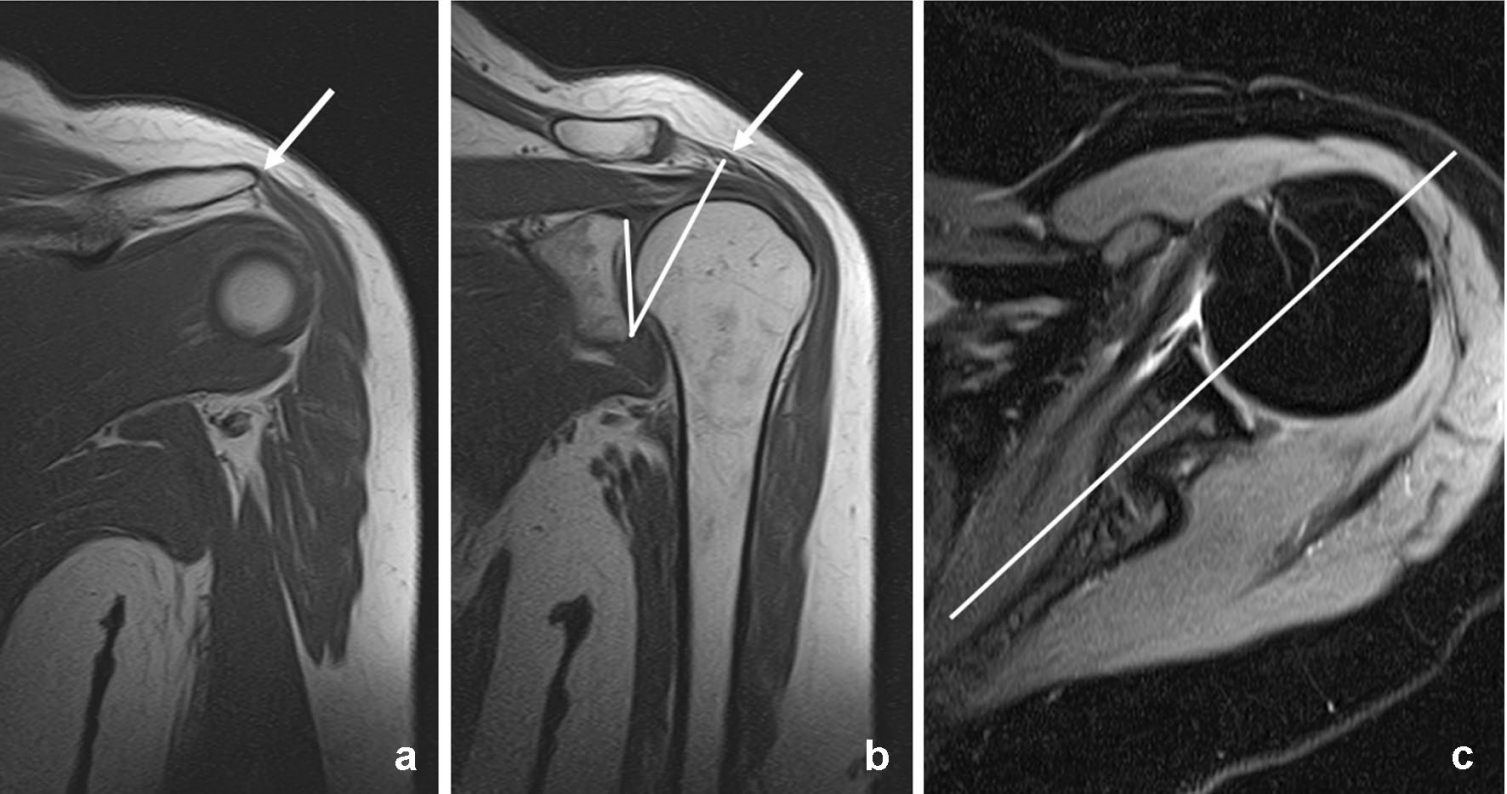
Measurement of the critical shoulder angle. T1-weighted images in a coronal plane a, b. Transversal proton-density weighted fat saturated images c. The most lateral extent of the acromion is marked in a coronal plane (*arrow* in a). After scrolling to a coronal plane, representing the middle of the glenoid b, the critical shoulder angle was measured. The white line in c represents the imaging plane of b
